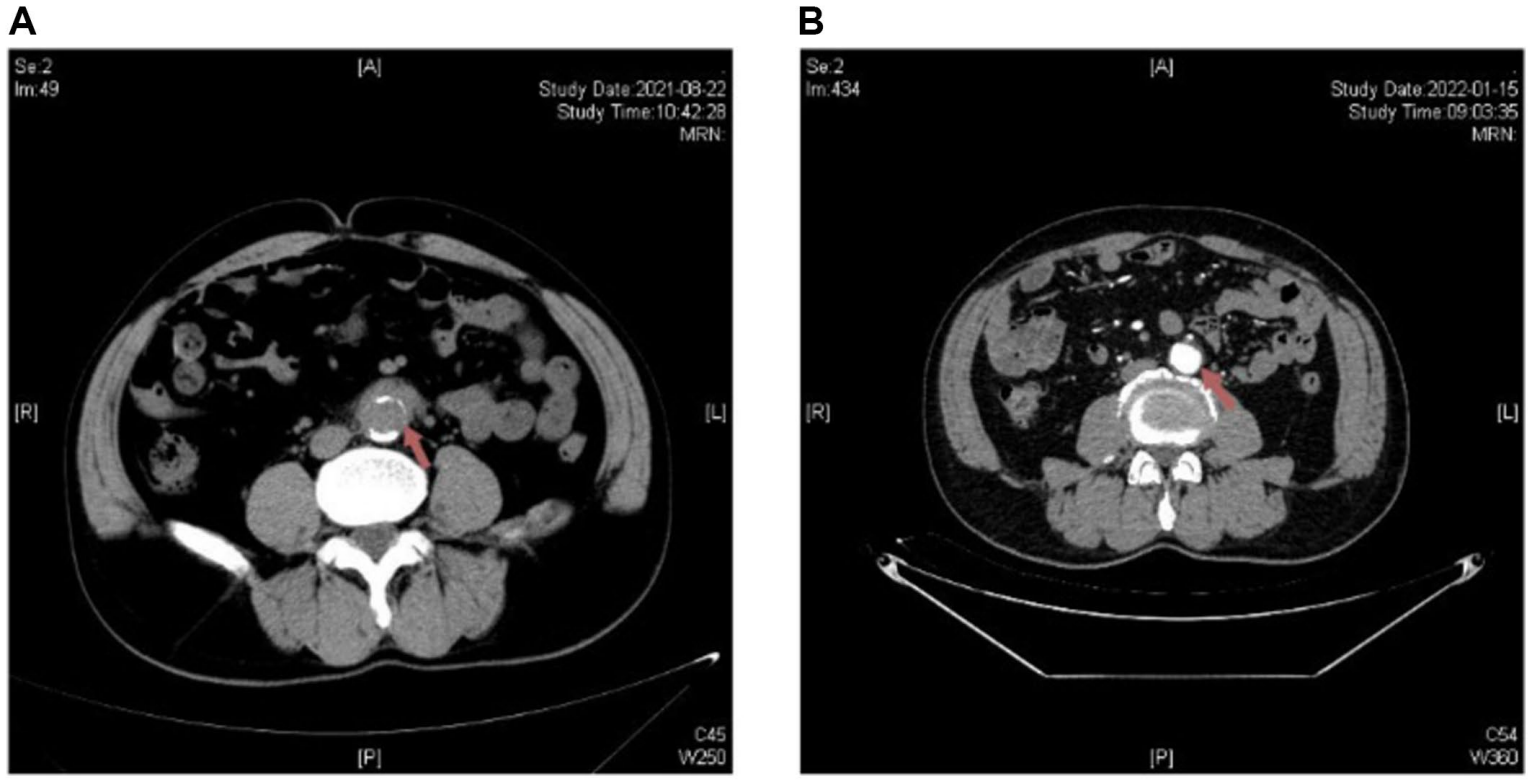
Radiological changes on abdominal aortic CTA before and after antibiotic treatment in Patient 3. (A) Upon admission: the distal end of the abdominal aorta is encircled by soft tissue density. (B) After treatment: the wall of the distal portion of the abdominal aorta shows uneven thickening, with significant improvement compared to the previous examination.
